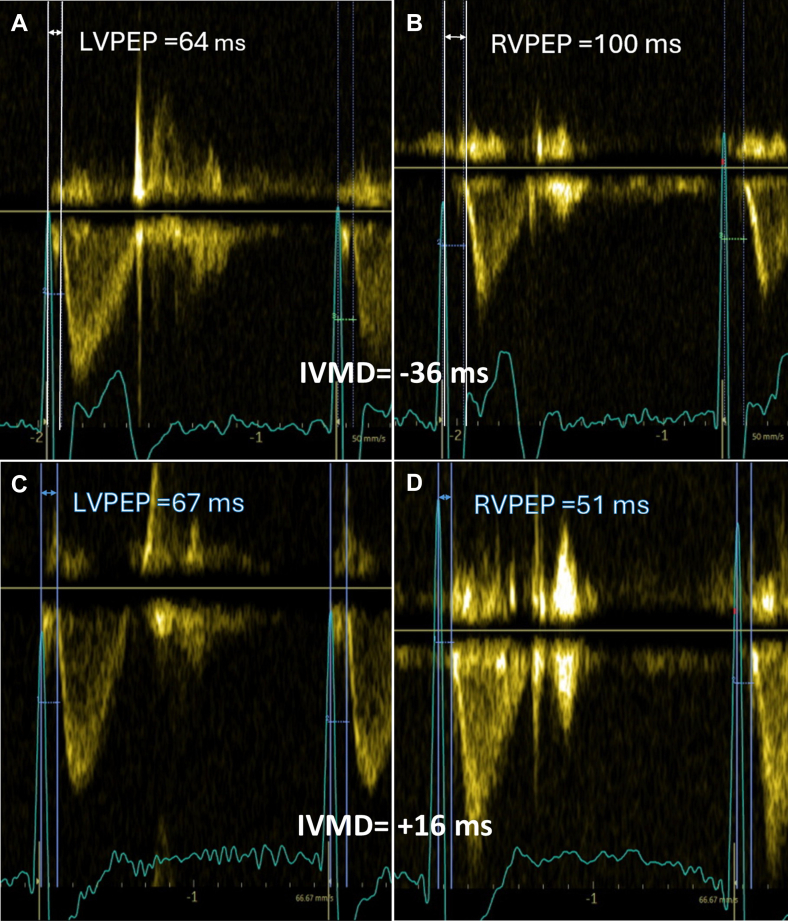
Interventricular mechanical delay (IVMD) before and after right ventricular-cardiac resynchronization therapy implantation. Pulsed-wave Doppler tracings showing LV pre-ejection period (LVPEP) and right ventricular pre-ejection period (RVPEP) with caliper measurements. A–B: pre-implantation; C–D: post-implantation. IVMD = LVPEP − RVPEP.
